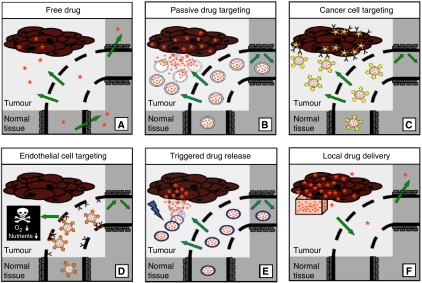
Overview of the clinically most relevant drug targeting strategies. (A) Upon the intravenous injection of a low-molecular-weight (chemo)therapeutic agent, which is often rapidly cleared from blood, only low levels of the drug accumulate in tumours and in tumour cells, whereas their localisation to certain healthy organs and tissues can be relatively high. (B) Upon the implementation of a passively targeted drug delivery system, by virtue of the enhanced permeability and retention (EPR) effect, the accumulation of the active agent in tumours and in tumour cells can be increased substantially. (C) Active drug targeting to internalization-prone cell surface receptors (over)expressed by cancer cells generally intends to improve the cellular uptake of the nanomedicine systems, and can be particularly useful for the intracellular delivery of macromolecular drugs, such as DNA, siRNA and proteins. (D) Active drug targeting to receptors (over)expressed by angiogenic endothelial cells aims to reduce blood supply to tumours, thereby depriving tumour cells from oxygen and nutrients. (E) Stimuli-sensitive nanomedicines, such as Thermodox, can be activated (i.e., induced to release their contents) by externally applied physical triggers, such as hyperthermia, ultrasound, magnetic fields and light. (F) In cases in which tumours are easily accessible, for example during surgery, sustained-release delivery devices can be implanted or injected directly into (the irresectable parts of the) tumours.
